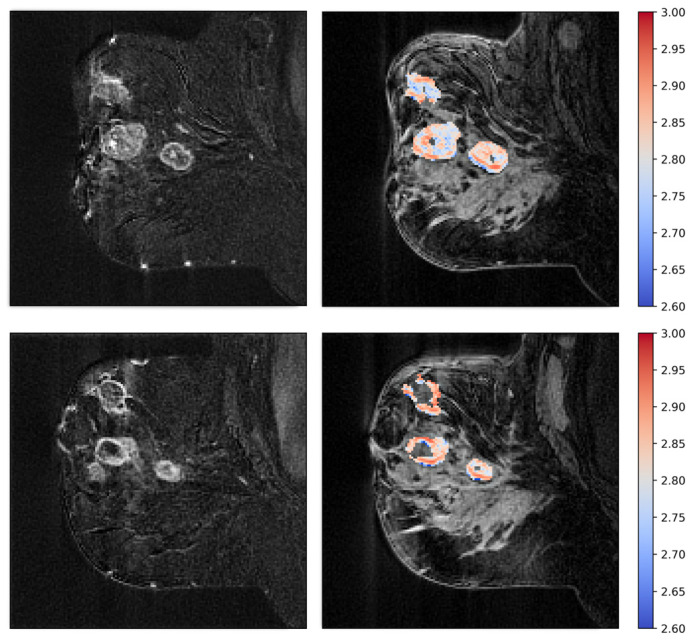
Patient treated with bevacizumab showing intermediate pre-treatment fractal dimension (top), with a significant increase in fractal dimensionality after one week of treatment (bottom). The fractal dimension maps are shown to the right as colored overlays on T2-weighted images. To the left are the subtraction images that the fractal dimension is calculated from.
